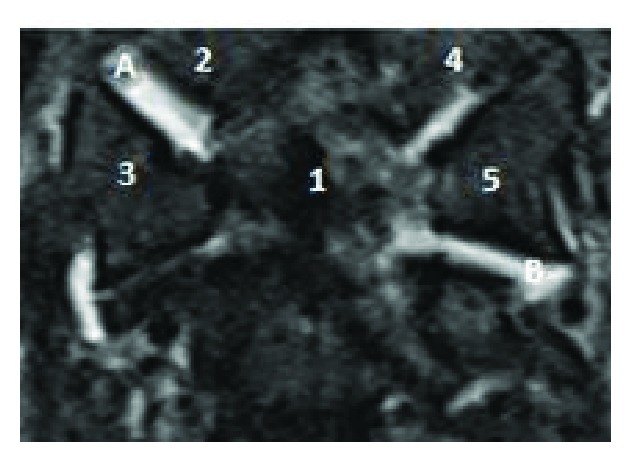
Cervical MRI coronal view. Dens (1), right occipital condyle (2), right C1 lateral mass (3), left occipital condyle, left C1 lateral mass. Note the bilateral edema in the right atlantooccipital joints (A) and the edema in the C1–C2 reflect mild capsular disruption.
